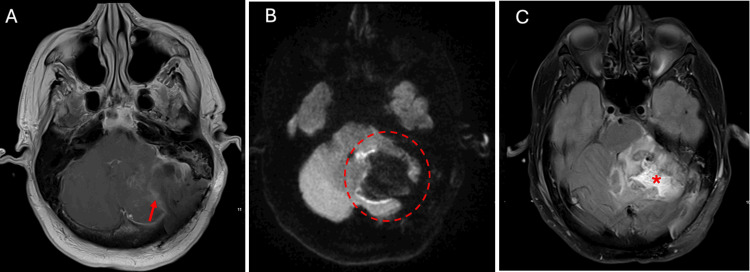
The patient's postoperative brain magnetic resonance imaging (MRI) T1-weighted post contrast axial MRI demonstrating gross total resection (panel A, red arrow) with minimal residual blood products. Diffusion-weighted imaging (DWI) showing resection cavity with no evidence of acute postoperative stroke (panel B, red circle). T2 Fluid-attenuated inversion recovery (FLAIR) axial MRI showing post-op edema (panel C, red asterisk) causing significant mass effect on the brainstem, which is stable compared to the preoperative scan.
